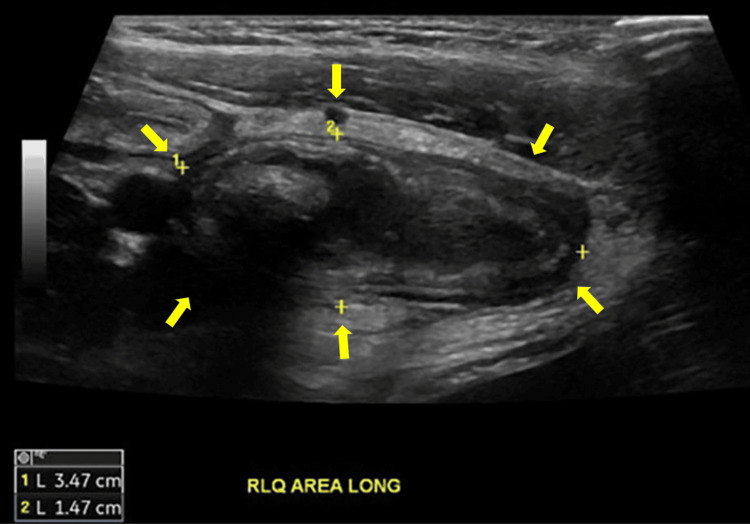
Ultrasound image with arrows pointing to an enlarged appendix with a probable appendicolith.
